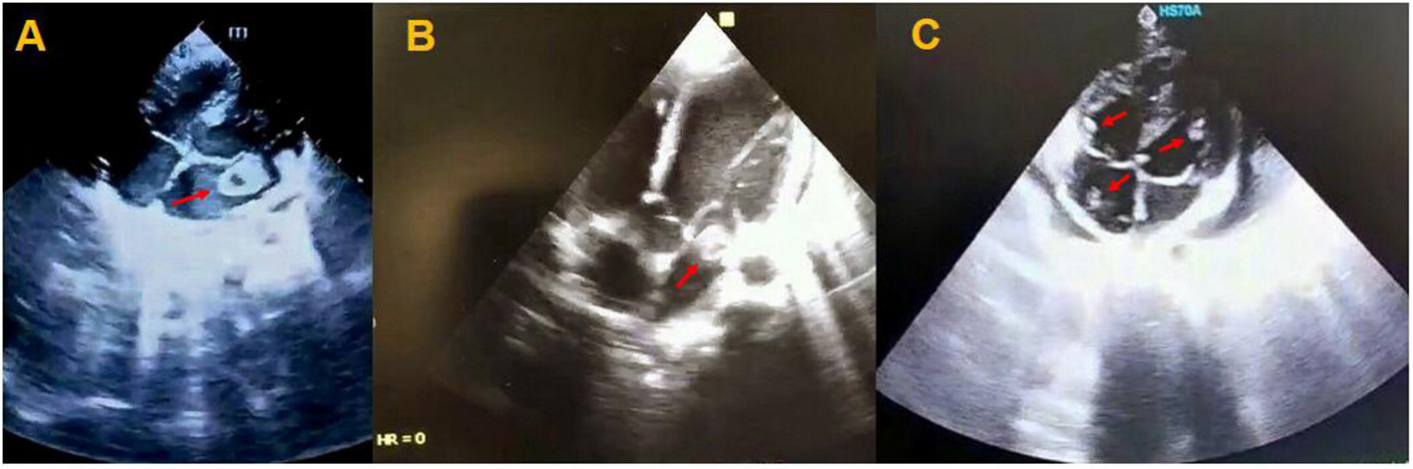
Transthoracic echocardiogram of patients showing a mobile mass in the left atrium with attachment to the posterior leaflet of the mitral valve (A), a large mobile mass in the left atrium (B), and multiple mobile homogenous masses in the right side of the heart and left ventricle (C).
